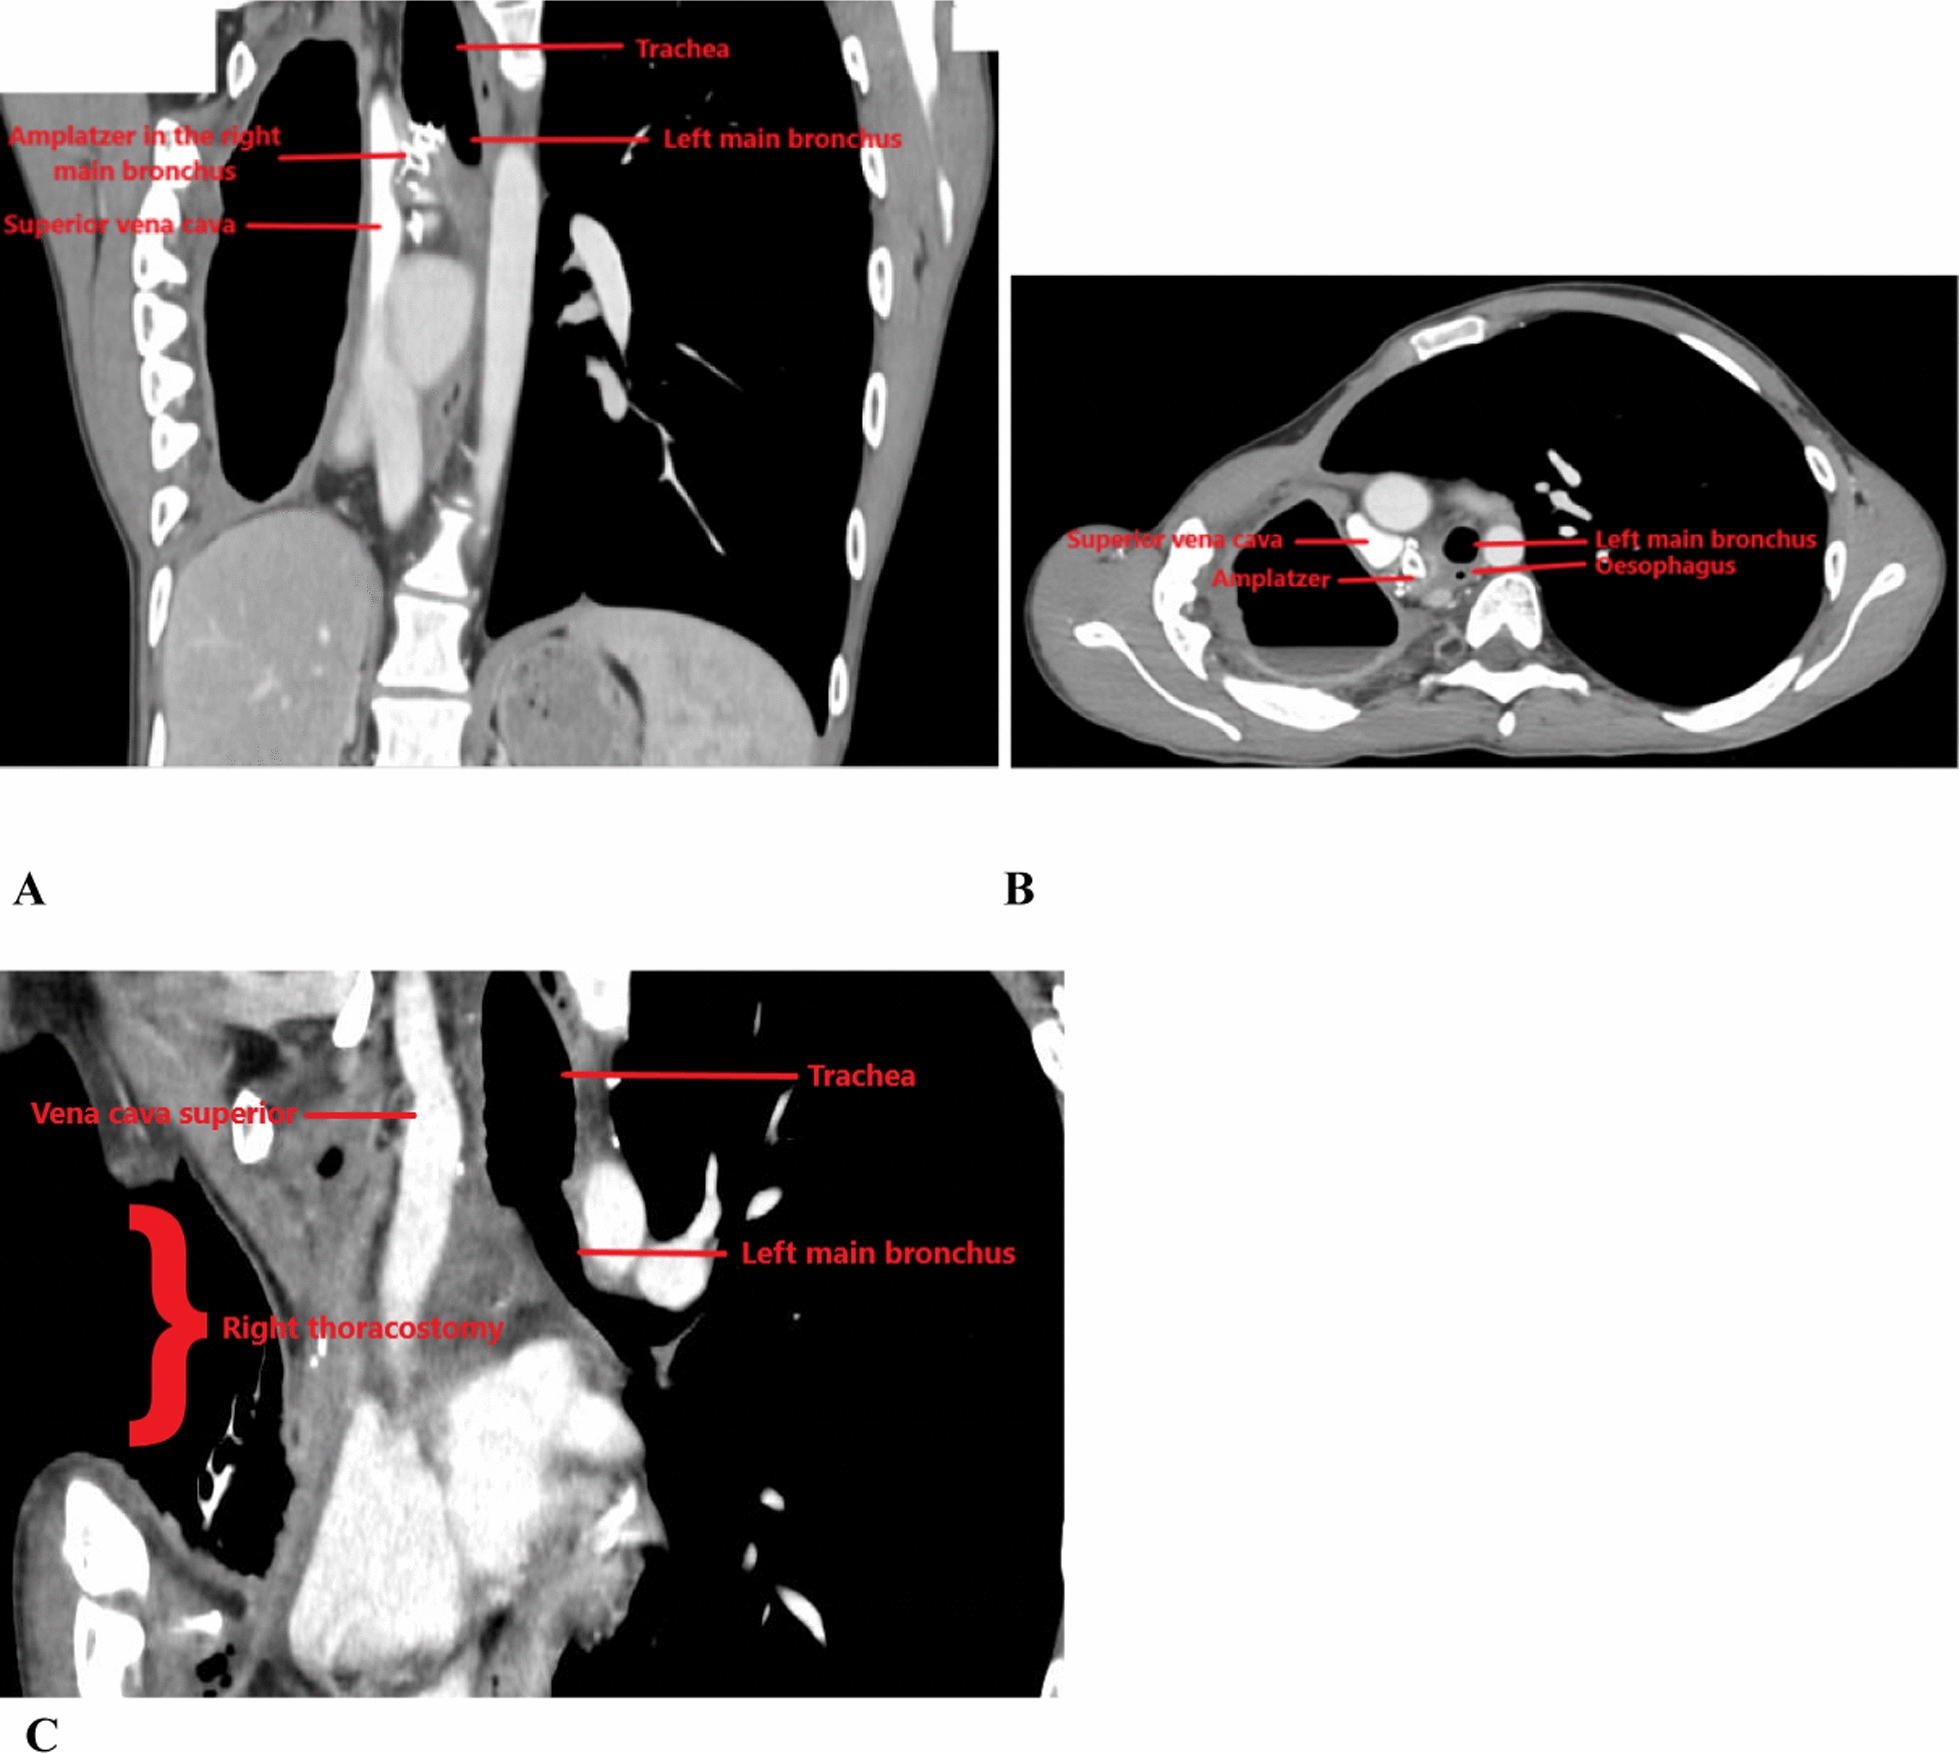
Computed tomography findings: 2A Coronal view before surgery; 2B Axial view before surgery; 2C Coronal view after surgery
